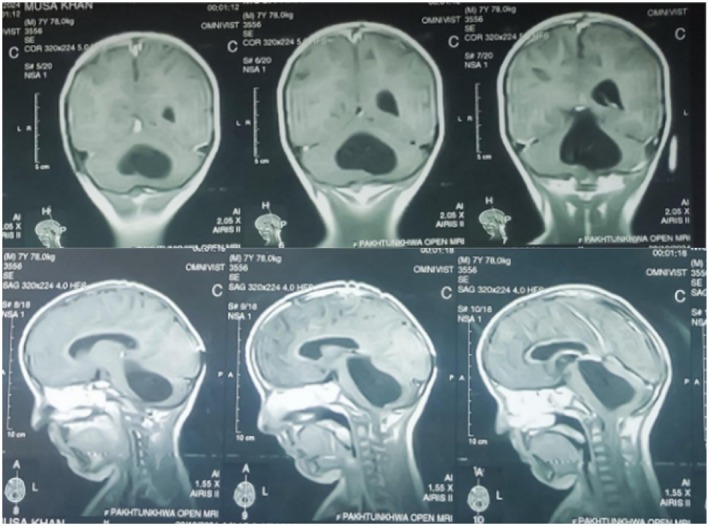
Pre‐operative MRI Brain showing hypointense lesion in the posterior cranial fossa on sagittal and coronal sections.
